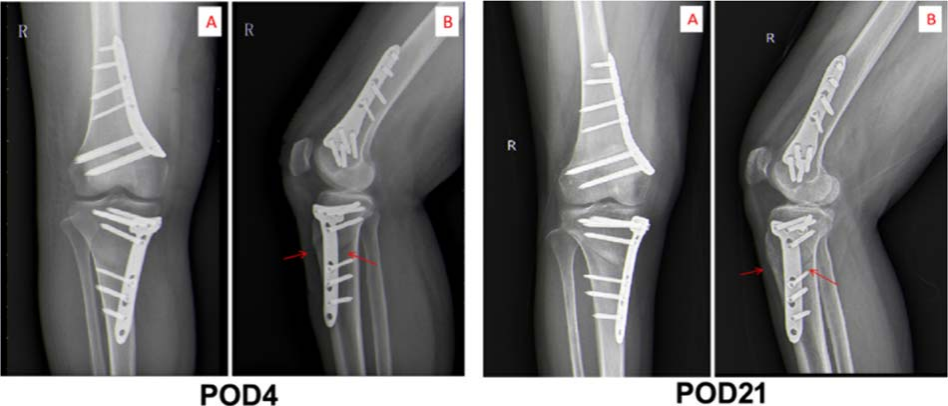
Computed axial tomography of chest. The images of CT scan on POD4 and POD21.
